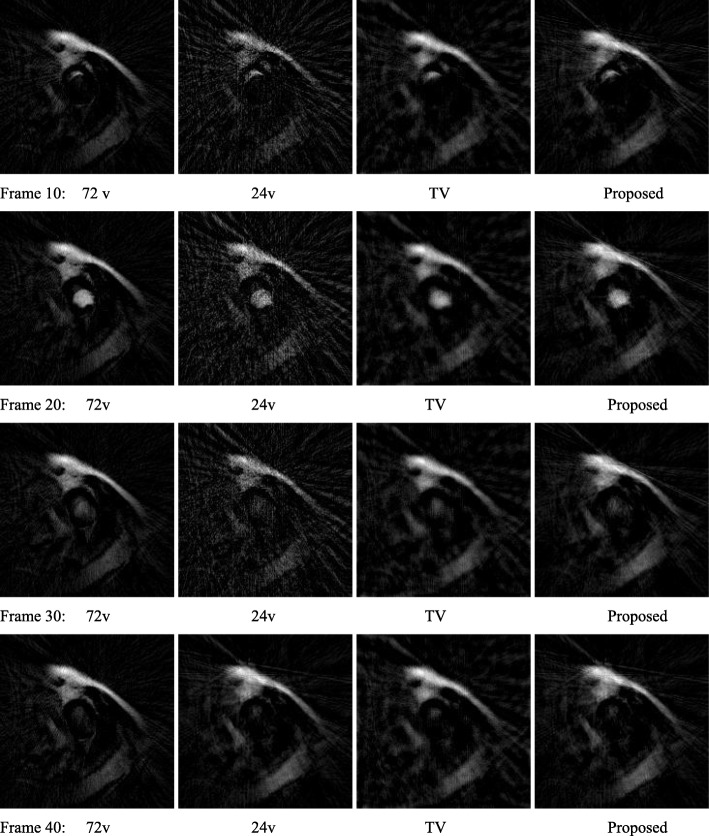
(Frame 10 ~ 40). MRI patient image reconstructions by using (1st column) 72-view FBP, (2nd column) 24-view FBP, (3rd column) 24-view iterative TV, and (4th column) 24-view proposed method. The 1st column is treated as the gold standard for other columns to compare with. The four rows of images correspond to time frames of 10, 20, 30, and 40, respectively
